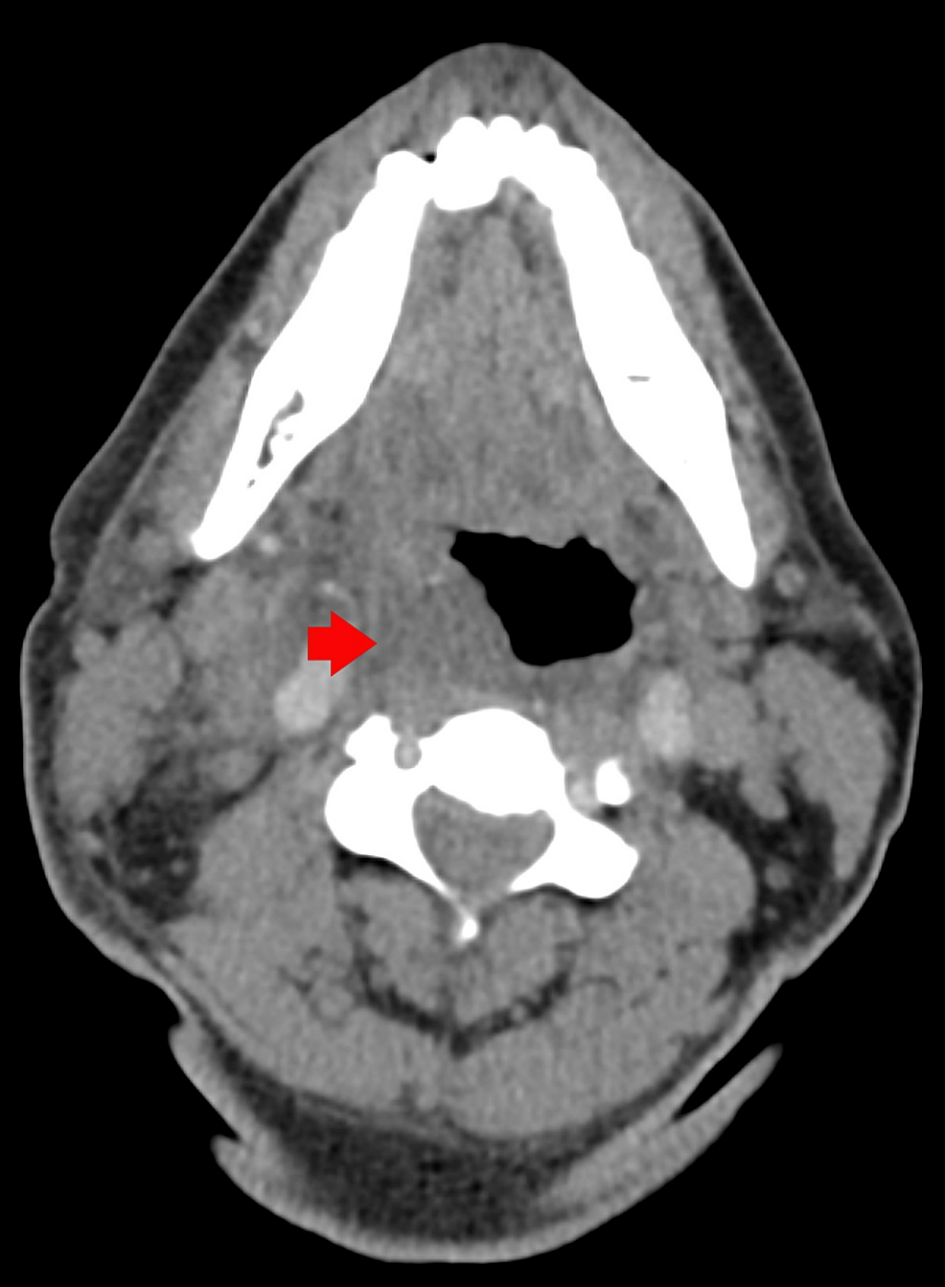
Computed tomography of the neck and soft tissue showing peritonsillar edema and possible abscess formation (red arrow)
